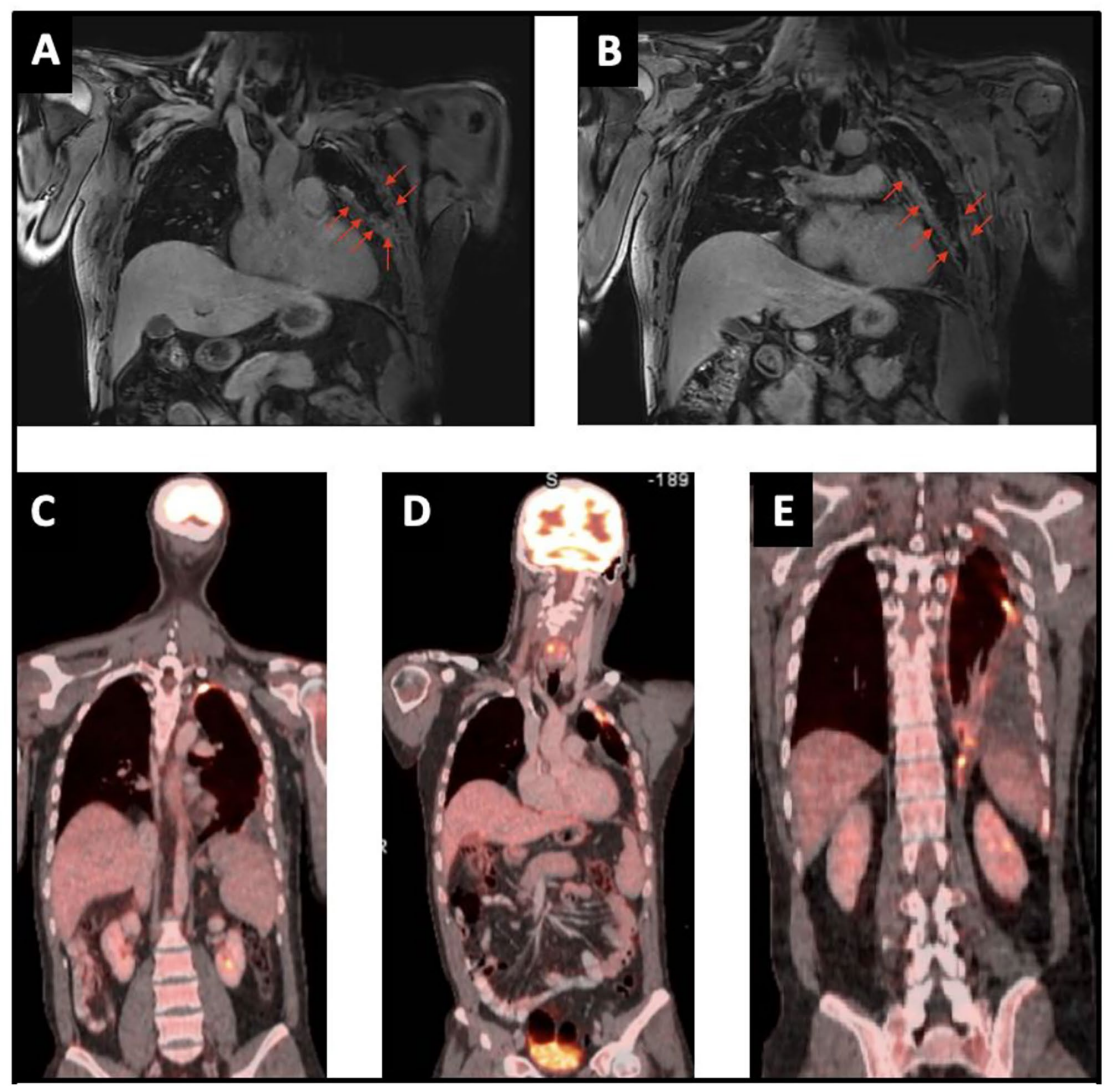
(A, B) Preoperative chest MRI showing residual pleural disease after chemotherapy; (C, D, E) Preoperative thoracic PET-CT showing residual active pleural disease after chemotherapy
